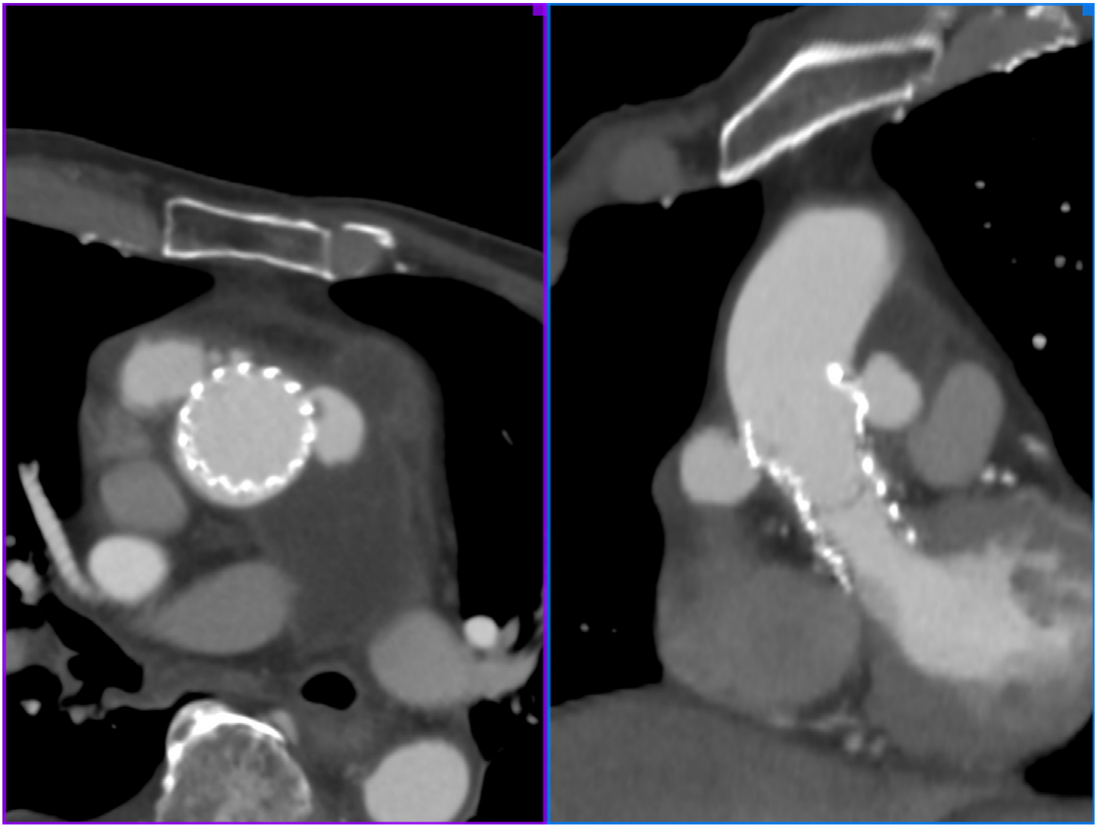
Multiplanar reconstruction views of computed tomography on admission. Two pseudoaneurysms are visualized on both sides of the ascending aorta near the distal stent site, and a massive hematoma is also visualized around the pseudoaneurysm.
